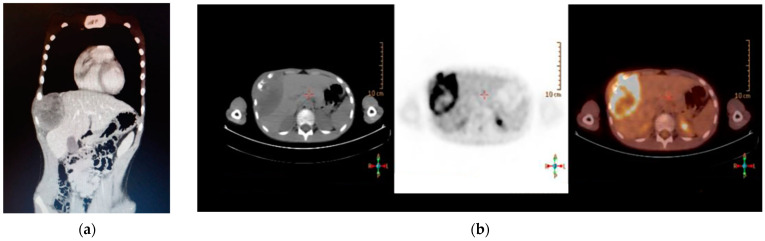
Images at presentation. (a) CT scan; (b) PET scan.
